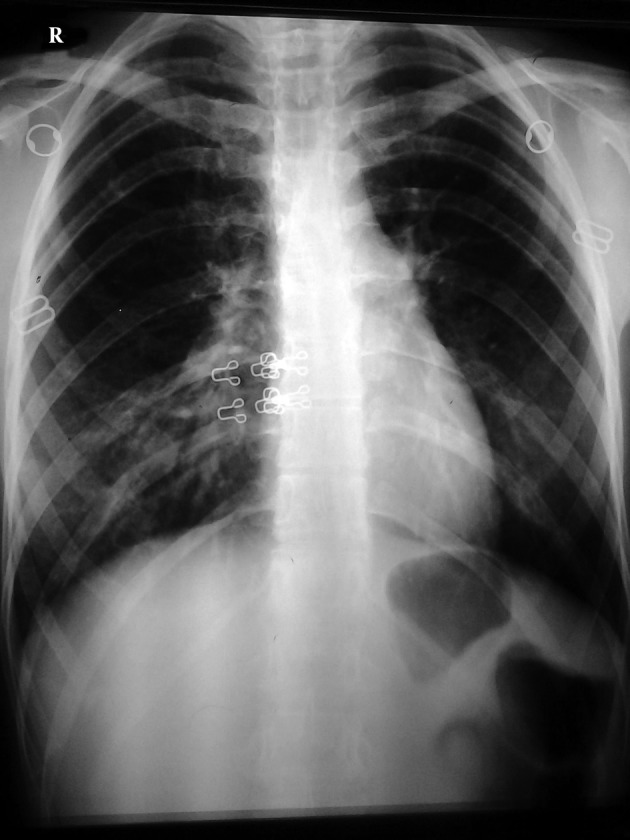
Posteroanterior chest radiograph shows patchy ill-defined infiltrates
